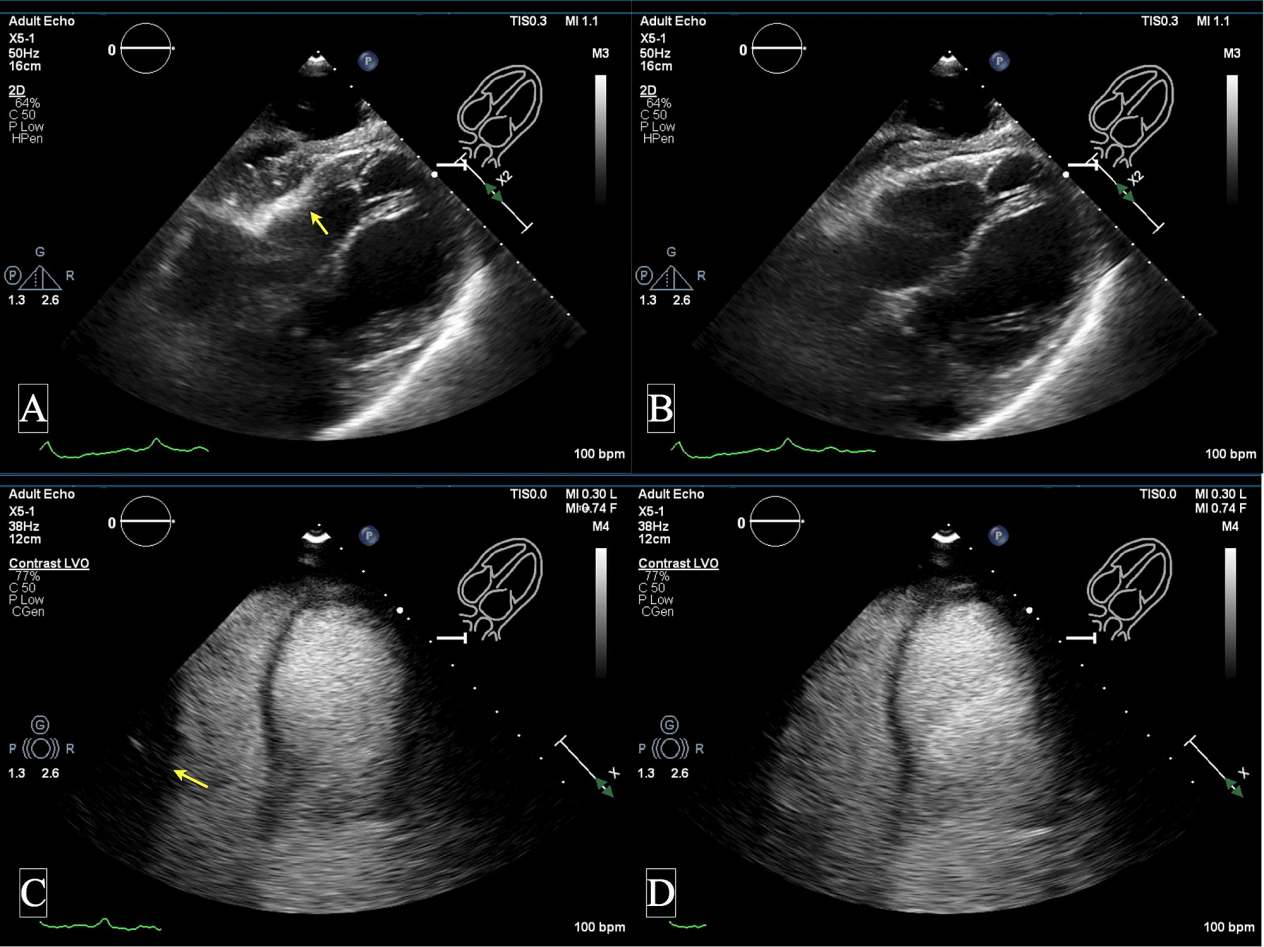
TTC: subcostal view without contrast and apical view with contrast Images are showing subcostal view without contrast (A-B); apical four-chamber view with contrast (C-D) showing biventricular takotsubo with apical akinesias and hyperdynamic basal segment (marked by the yellow arrow). TTC - takotsubo cardiomyopathy
